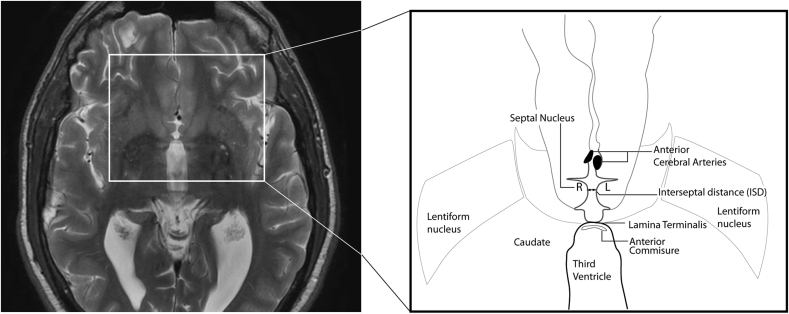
Axial T2-weighted image of an adult brain demonstrating anatomical landmarks for ISD measurement.
